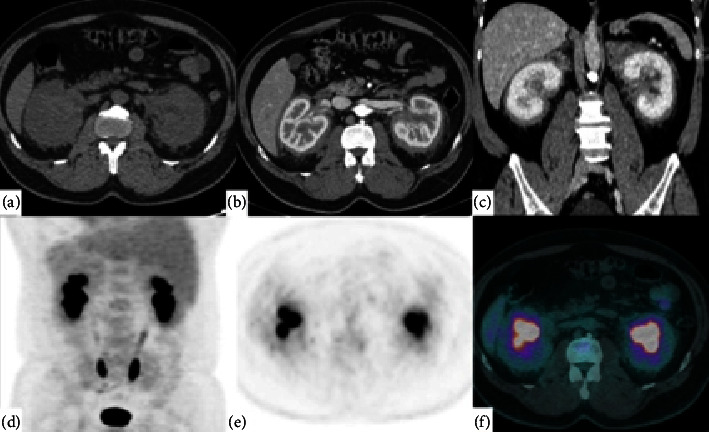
Computed tomography images of the abdomen: (a) before administration of the intravenous iodinated contrast, (b) corticomedullary phase, and (c) coronal reformatted image of the nephrographic phase, showing tissue with soft-tissue density surrounding the renal parenchyma and infiltrating the renal pelvis bilaterally. Note the slight dilatation of the bilateral collecting system resulting from extrinsic compression by the tissue. Positron emission tomography/computed tomography (PET-CT): no radiopharmaceutical uptake is seen on PET-CT as shown in (d) coronal PET, (e) axial PET, and (f) fusion of PET with tomographic image. Percutaneous biopsy confirmed the hypothesis of renal histiocytosis (Erdheim–Chester disease).
